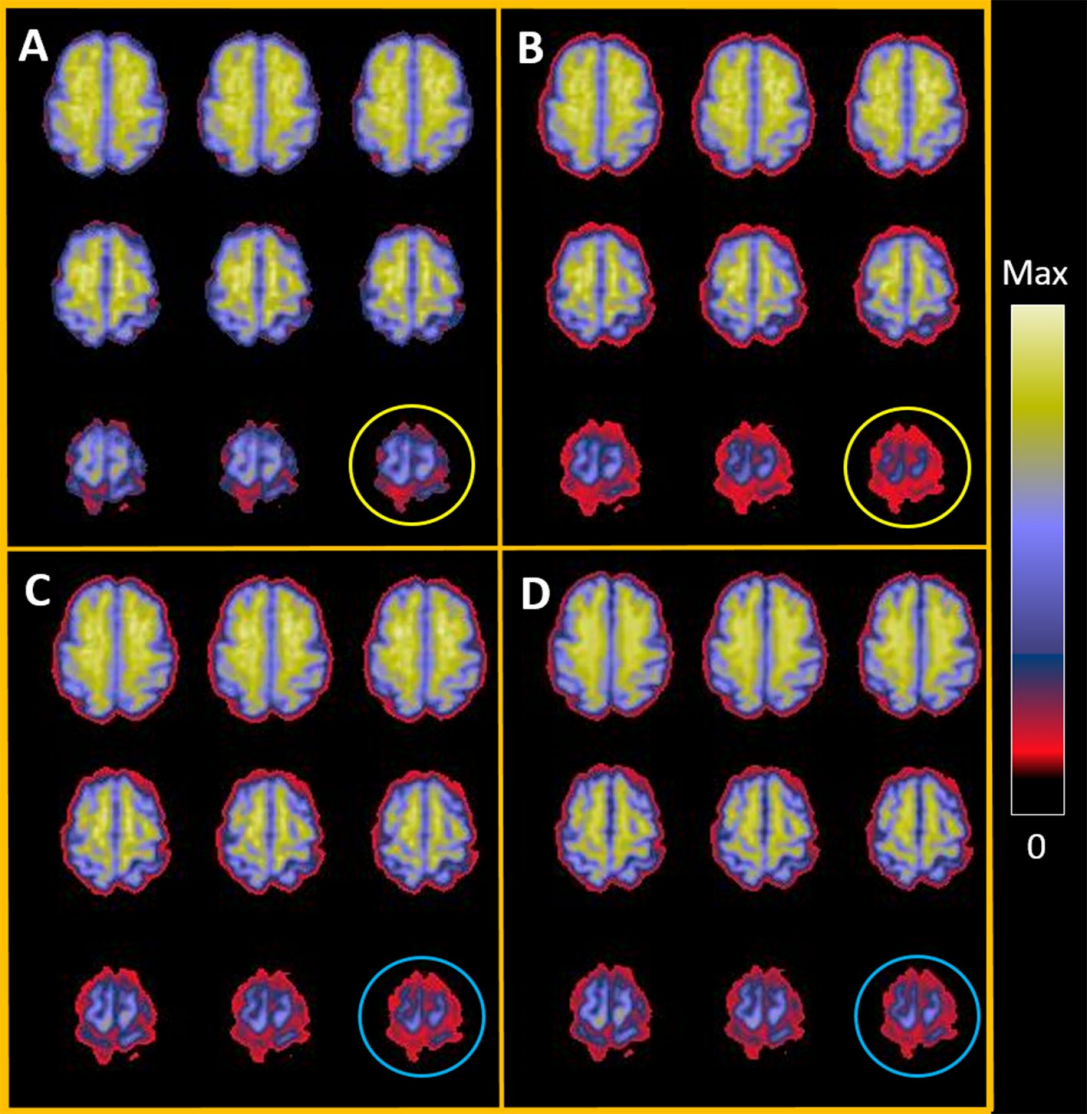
Sample images of PET data, coregistration, and PET models. Images show (A) PET data after rigid coregistration of native PET data to MRI space; (B) initial MRI-based model of PET data derived from FreeSurfer parcels, using values from (A) as inputs, with no PVC; (C) nonlinear coregistration of native PET data to MRI space; and (D) final MRI-based PET model using input values from (C) and incorporating PVC. The correspondence between (A) and (B) was grossly adequate but insufficient for details close to the cortical ribbon. Differences are best appreciated along the margins of features (e.g., near the apex, as shown in the orange circles). The correspondence between (C) and (D) was greatly improved with the use of nonlinear coregistration and PVC (see blue circles). The colored display reflects the SUVR, with the normalized magnitude indicated by the color bar, scaled between the maximum value (which was close to SUVR = 3.0) and zero. *MRI* magnetic resonance imaging, *PET* positron emission tomography, *PVC* partial volume correction, *SUVR* standardized uptake value ratio.
